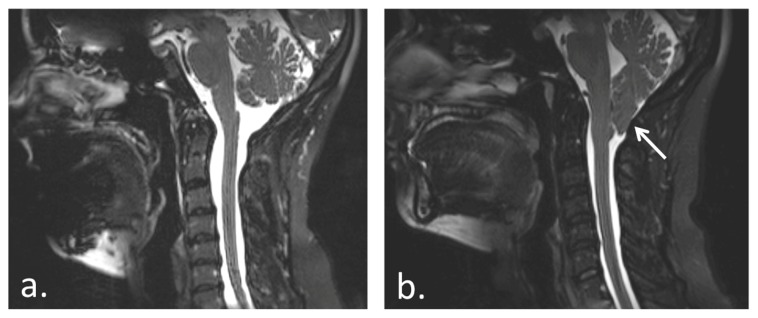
T2-weighted mid-sagittal MRI scan of (a) a healthy subject (b) and Chiari Type I malformation patient with arrow indicating location of tonsillar herniation through the foramen magnum and an asterisk indicating the medullary (brainstem) compression.
